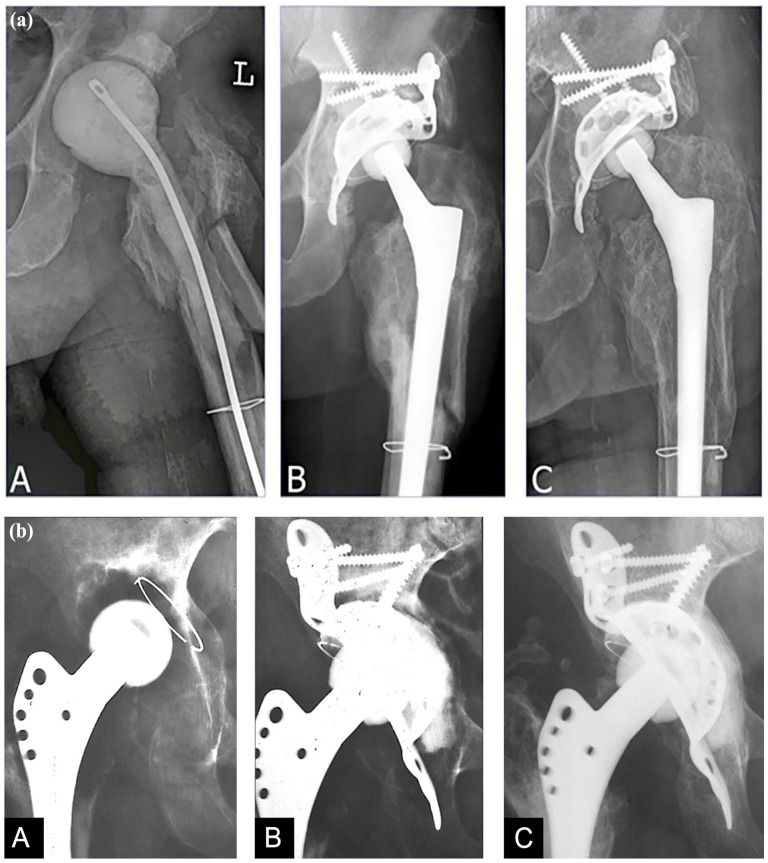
(a) Case description of a 76-year-old male patient. (A) 2-stage revision for periprosthetic joint infection with a cement spacer implanted. Intraoperative acetabular defect size AAOS 3. (B) Postoperative radiograph of implanted Burch-Schneider antiprotrusio cage, autologous bone graft, and femoral stem. Long screw pointing toward the iliosacral joint placed first. (C) Follow-up 11.2 years after revision showing no signs of loosening. (b) Case description of a 59-year-old female patient. (A) Massive loosening of a conic Endler polyethylene cup. Intraoperative acetabular defect size AAOS grade 4. (B) Following curettage, morsellised iliac crest autograft was placed cranially and medially in the cavity, and an BS-APC was implanted (C) Complete osseous integration without loosening or wear at 11-year follow-up.
